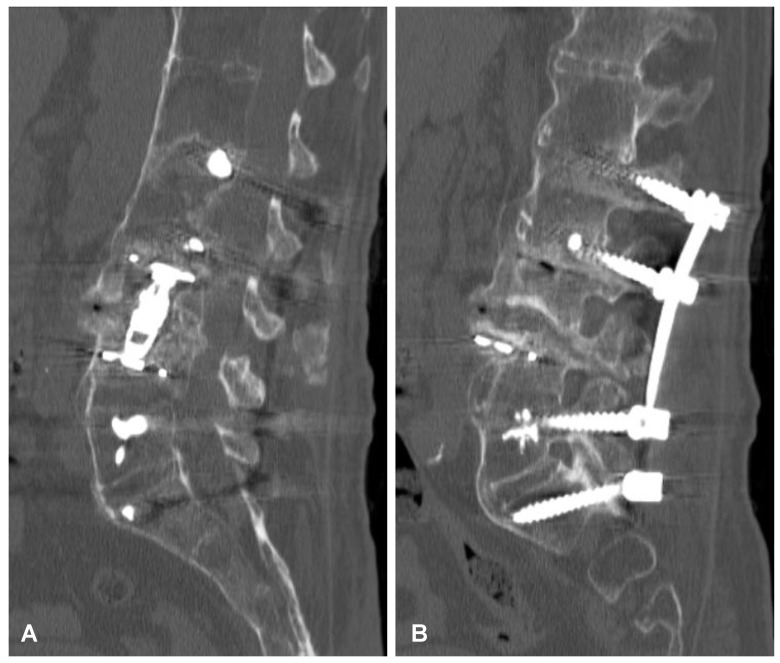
Postoperative sagittal CT scans (non-contrast enhanced) after the two-stage surgery of the lumbar spine demonstrating placement of the distractible vertebral body replacement implant between L3 and L4 (A) and posterior percutaneous transpedicular stabilization at the L2-L3-L5-S1 levels (B).
